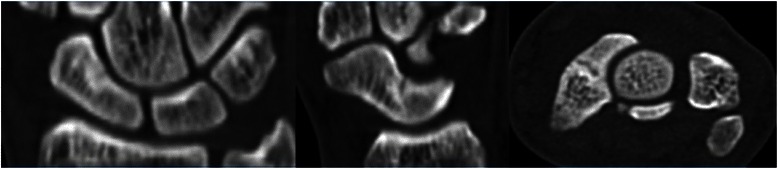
Computed tomographic image from the first visit shows a nondisplaced fracture at the scaphoid waist. Sclerotic and cystic changes suggest stress fractures of the scaphoid
